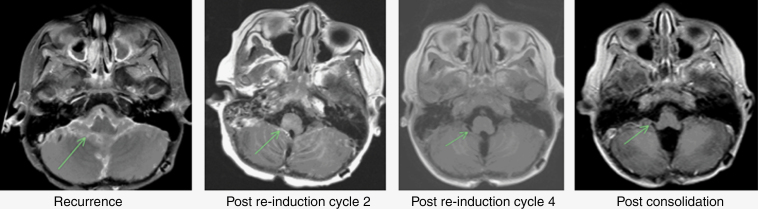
Representative MRI images from patient 2.
